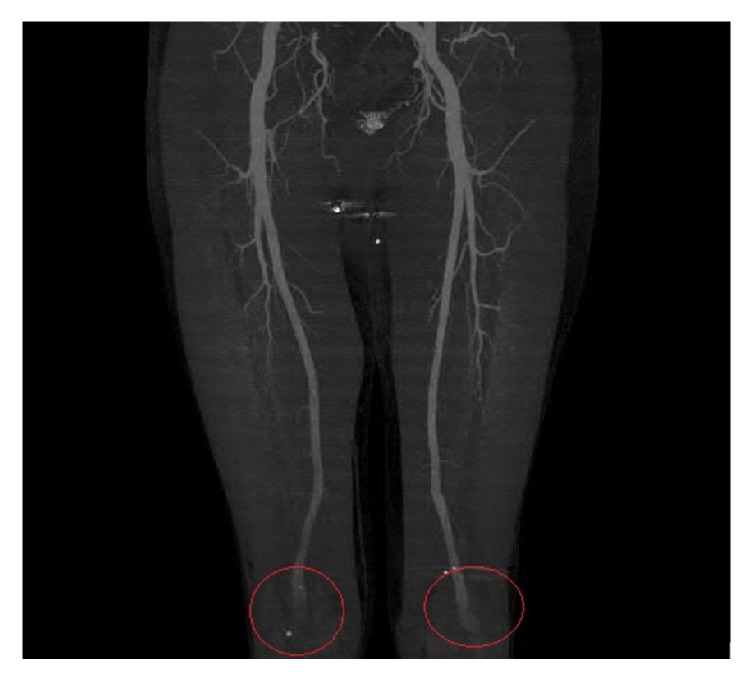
CT angiogram MIP images showing bilateral popliteal aneurysms highlighted by red circles.
